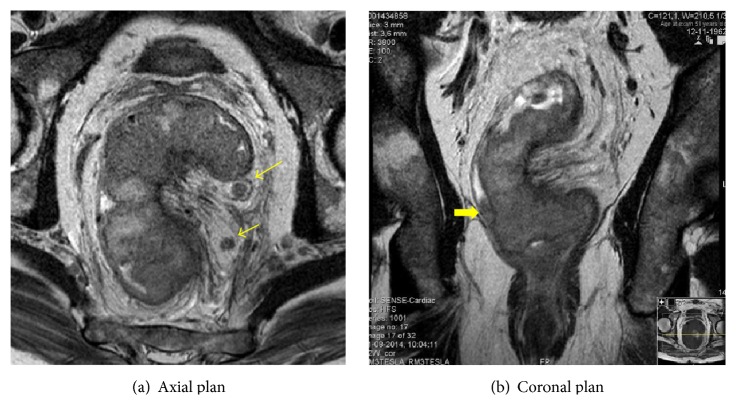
MRI before CRT. Large tumor with 9 cm of longitudinal length and 7 cm of transverse diameter that extends to left side of mesorectal fascia, multiple enlarged pelvic lymph nodes. Thin arrow: metastatic lymph node; thick arrow: mesorectal fascia invasion.
